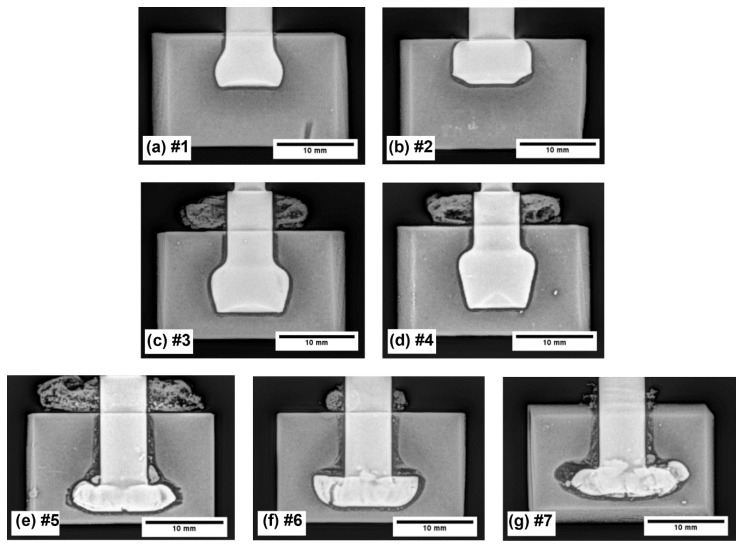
X-ray images of rivet shape and its position inside the base PEI material block (Note*: numbers (#1–#7) by the image captions (a–g) represent the sample designation using different manufacturing parameters—see Table 1).
